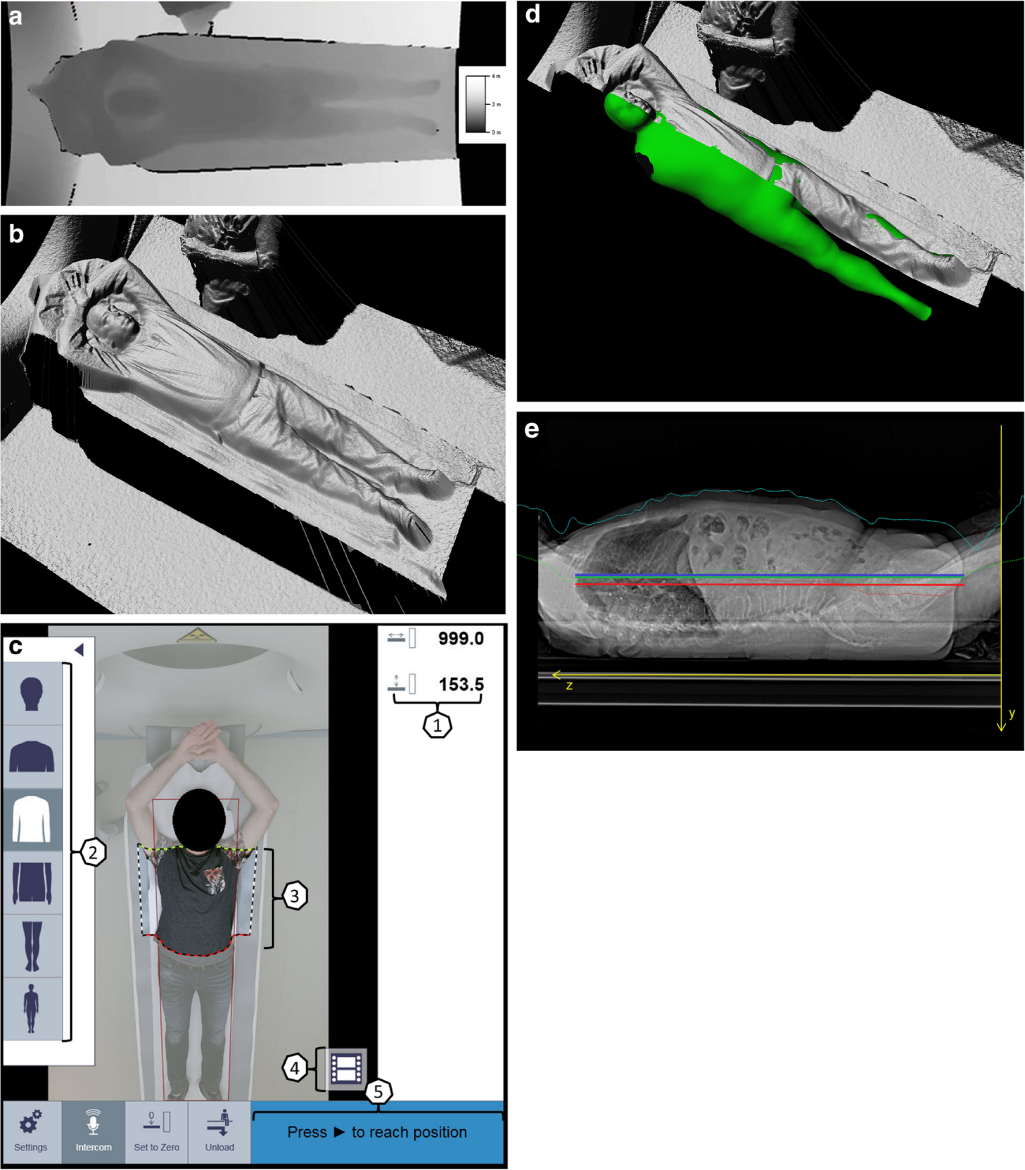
a Measured depth values. b Depth surface after perspective correction. c User interface of touch panel on CT scanner: 1, table position and table height; 2, selectable body region; 3, adjustable scan range; 4, taking planning image; 5, automatic position of the patient on base of selected scan range. d Virtual patient avatar. e Patient positioning accuracy: Red horizontal line: average patient isocenter, blue horizontal line: scanner isocenter, green horizontal line: average patient isocenter estimated by camera
